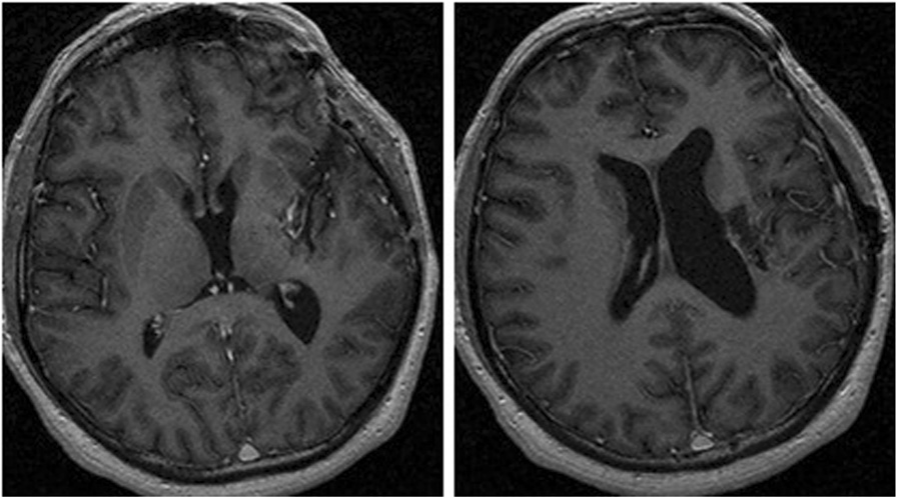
Control MRI (T1-enhancement) after 17 months of surgery. Post-surgical scar is presented in left insular region. No new intracerebral lesion
